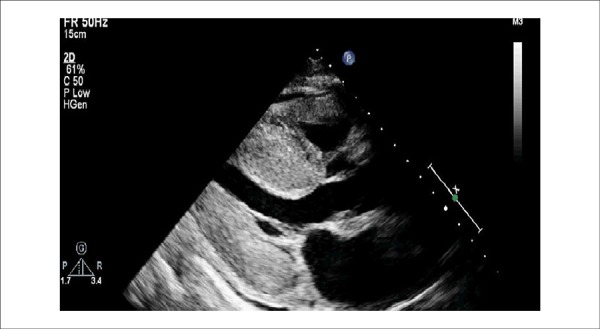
Echocardiography of cardiac amyloidosis with important left ventricular
